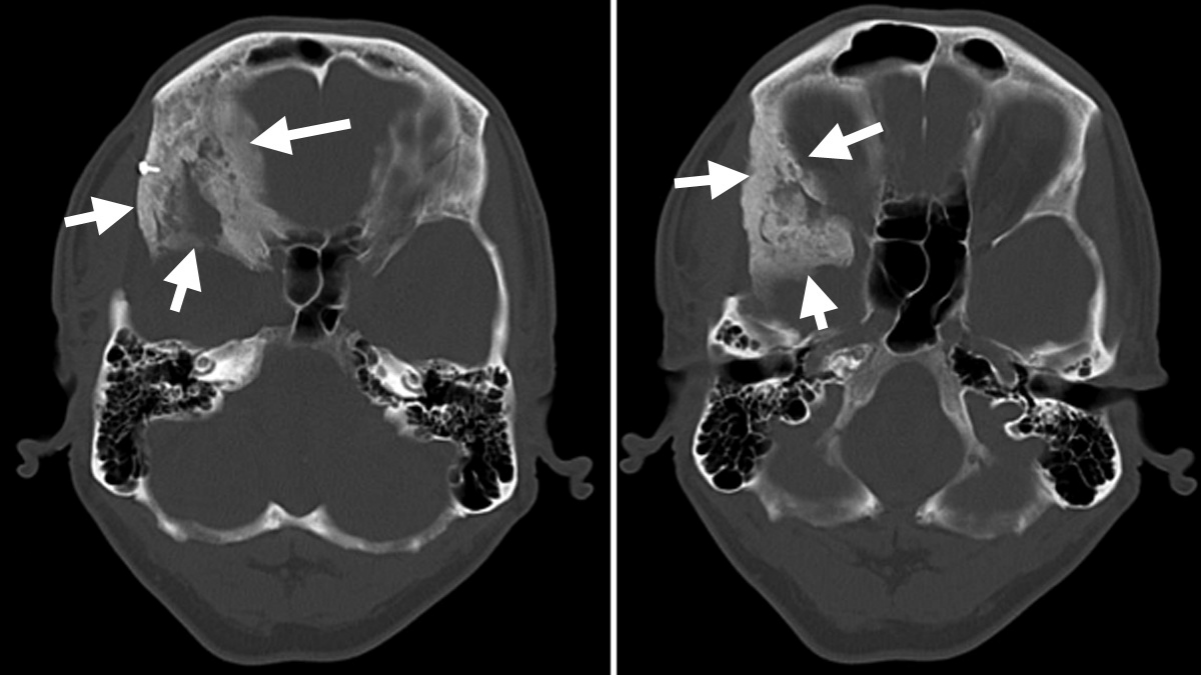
Preoperative computed tomography scan bone window images Axial cuts showing extensive hyperostosis of the lesser and greater sphenoid wing, lateral orbital wall, clinoid, and middle cranial fossa floor (arrows).
